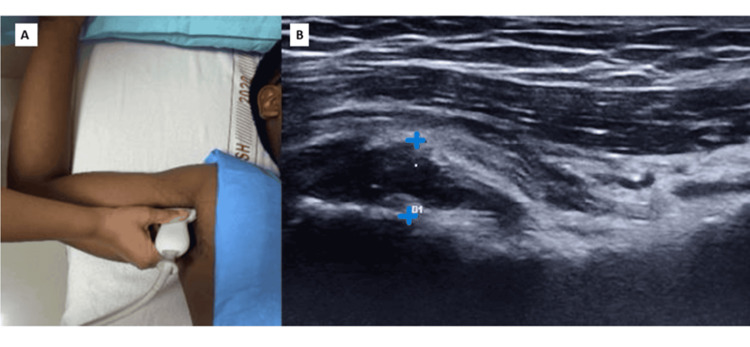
Measurement of axillary recess thickness by ultrasonography A) Position of the probe; B) Ultrasound image
